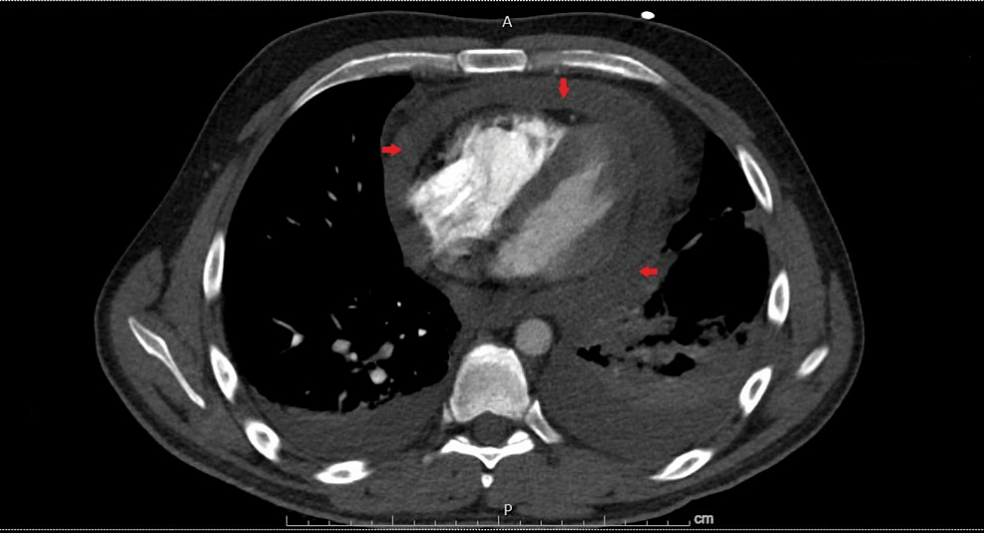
CT angiogram showing pericardial effusion (arrows) CT: computed tomography
